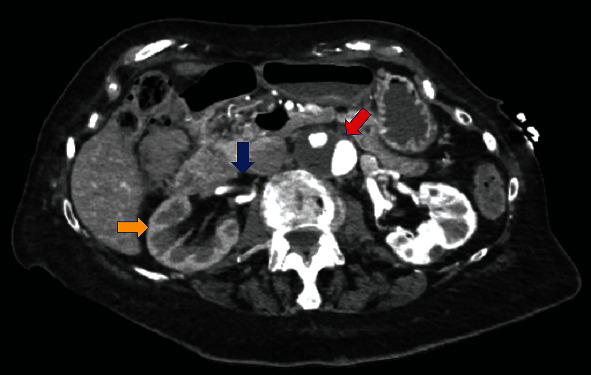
CTA of the aorta of the patient with bilateral run off showing infrarenal dominant abdominal aortic aneurysm with juxtarenal aortic dissection (red arrow); it also shows that the false lumen supplies the right renal artery which is mostly thrombosed (blue arrow), in addition to delayed right kidney nephrogram (yellow arrow).
